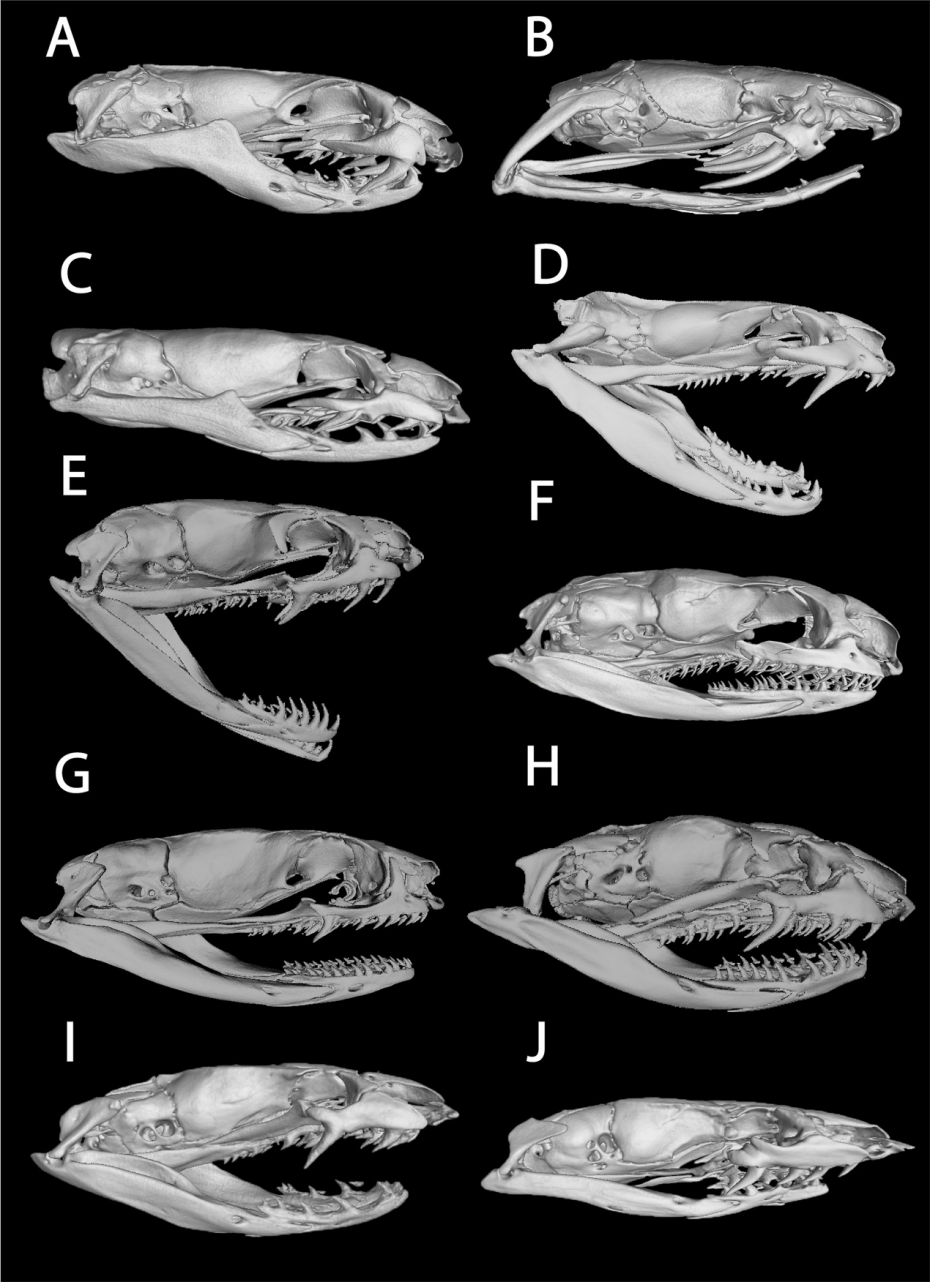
Computed tomography (CT) scans of aparallactine and atractaspidine genera. *Homoroselaps lacteus* (CAS 173258) (A); *Atractaspis bibronii* (CAS 111670) (B); *Chilorhinophis gerardi* (CAS 159106) (C); *Polemon christyi* (CAS 147905) (D); *Aparallactus niger* (AMNH 142406) (E); *Aparallactus modestus* (CAS 111865) (F); *Aparallactus capensis* (G); *Macrelaps microlepidotus* (H); *Amblyodipsas polylepis* (CAS 173555) (I); *Xenocalamus bicolor* (CAS 248601) (J).
